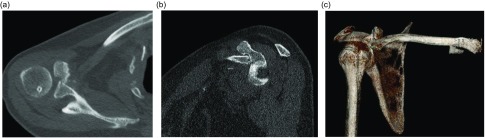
(a) Axial, (b) sagital, and (c) 3D computed tomography images of the right shoulder 8 weeks after injury demonstrating subcoracoid dislocation of the distal clavicle.
